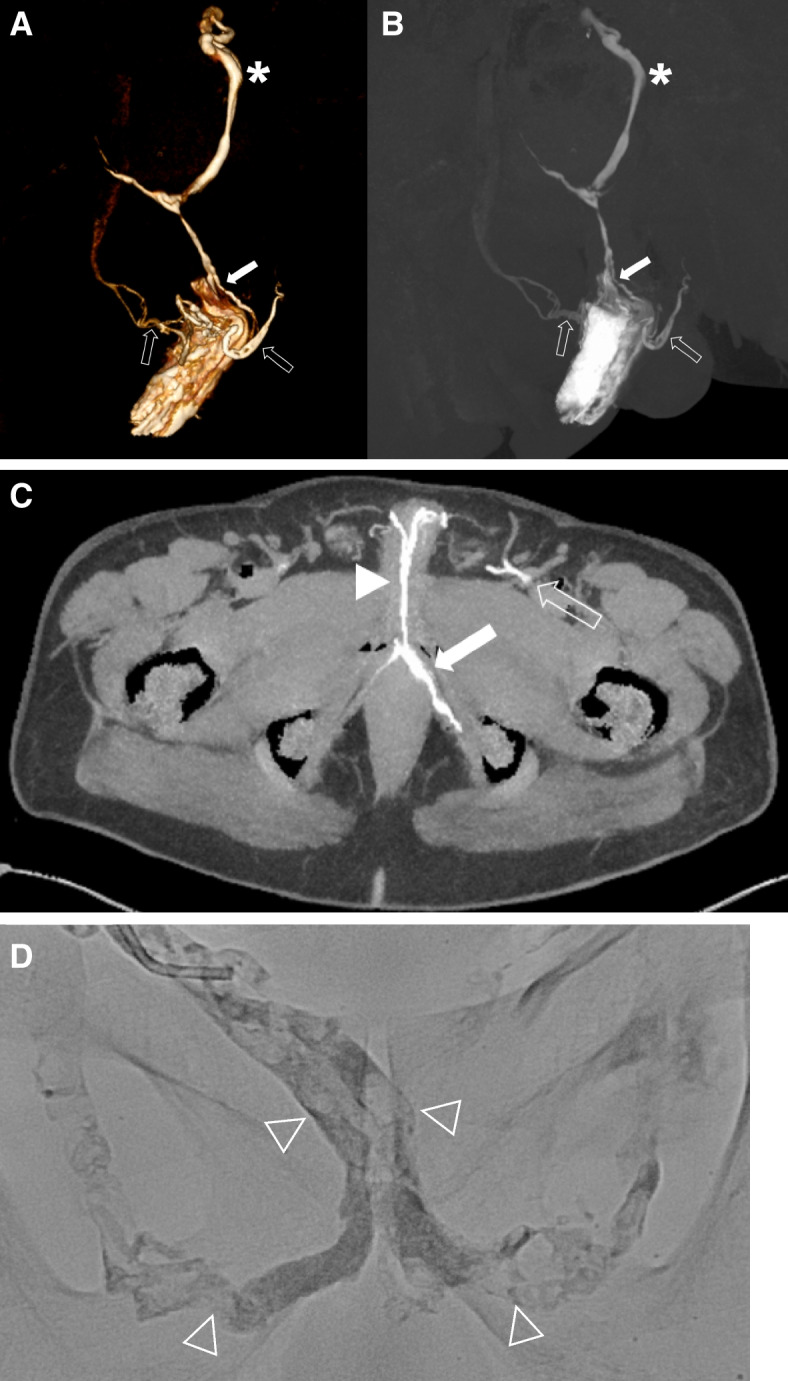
A 58-year-old patient with severe erectile dysfunction not responding to phosphodiesterase-5-inhibitors. CT-cavernosography depicting venous leaks of the deep dorsal penile vein (arrowhead), internal pudendal veins (arrows) draining into iliohypogastric veins (asterisk) and external (open arrows) pudendal veins on three-dimensional volume rendering (A), three-dimensional maximum intensity projection (B), and axial maximum intensity projection images (C). Image post venous leak embolization (D) with NBCA-based liquid embolic agent mixed with lipiodol (open arrowheads)
